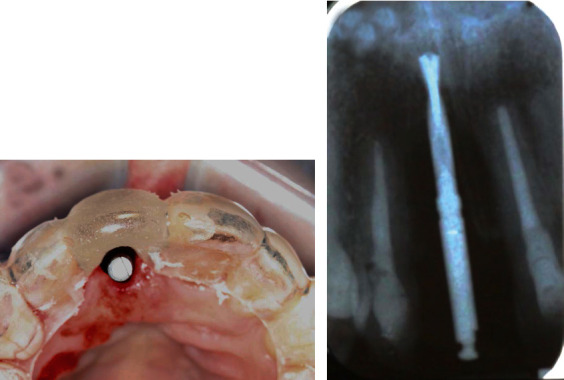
(a) Initial implant osteotomy was made at the cingulum region using a precision drill under the guidance of a surgical stent. (b) A periapical radiograph was taken with a 2 mm twist drill to verify the depth and angulation for implant placement.
